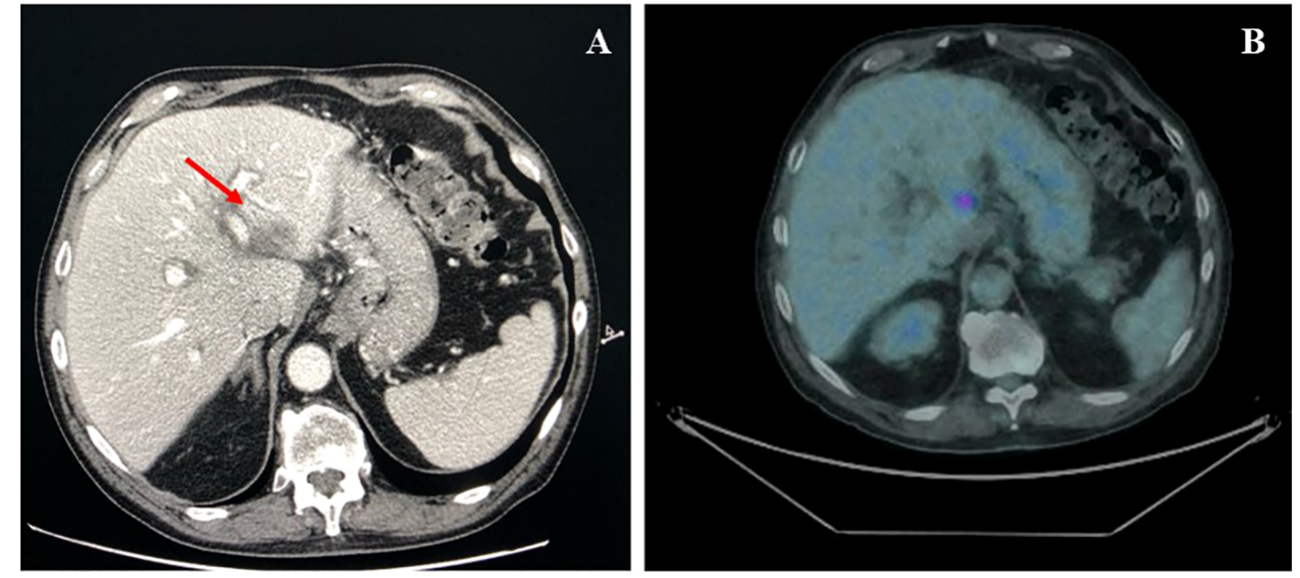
(A) Axial CT scan evaluation after 3 months of imatinib treatment (*arrow*), showing a partial response of liver lesion. (B) Axial PET/CT fused images scan, after 3 months of imatinib treatment, showing a marked decline in both lesion size and ^18^F-FDG uptake (SUVmax 7.9).
